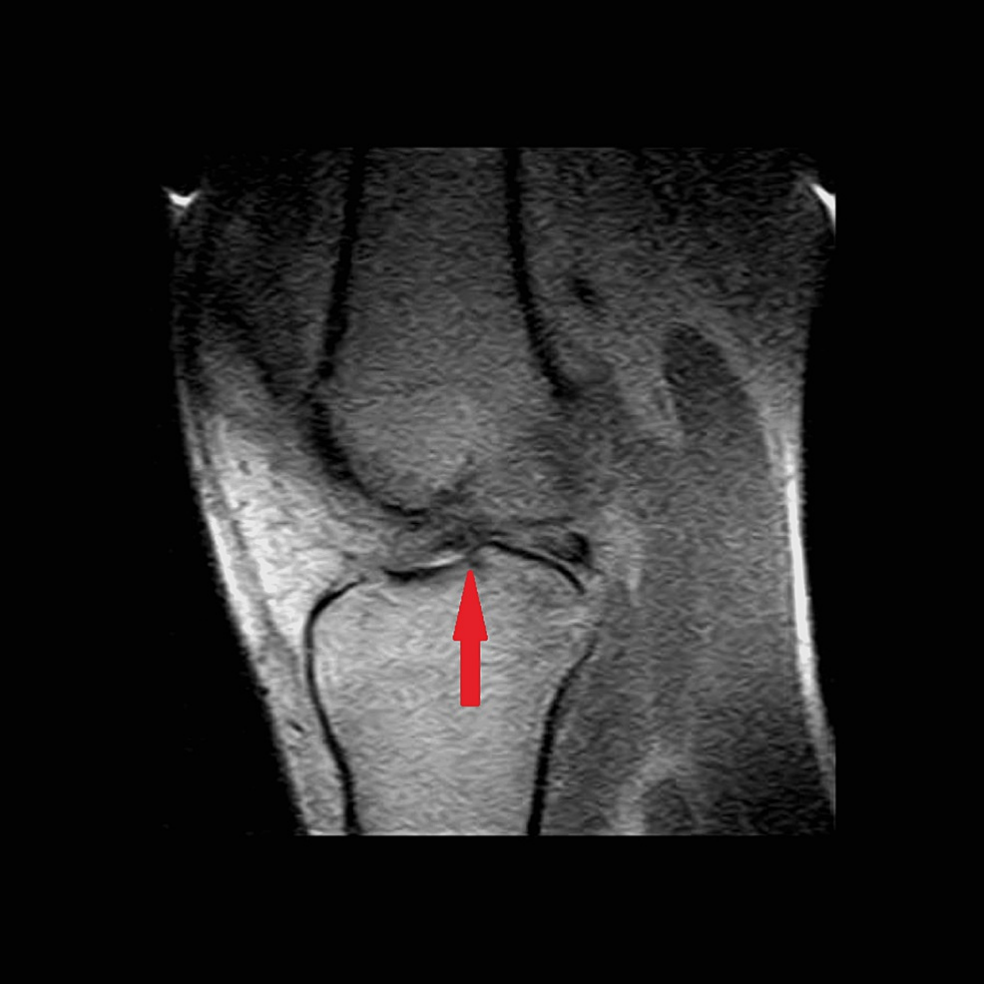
T1 sagittal MRI of the left knee. Red arrow points toward the evidence of inflammation. MRI: magnetic resonance imaging
